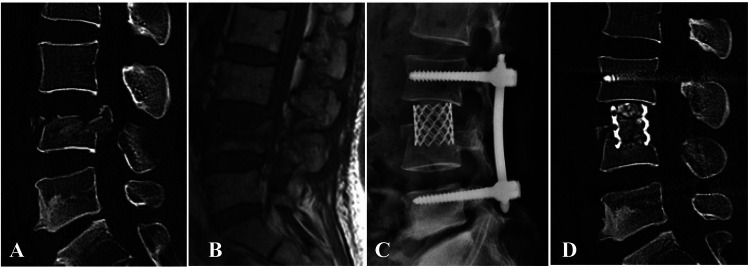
A patient with L4 burst fracture underwent posterior instrumentation combined with AIPA monosegmental reconstruction, in which the inferior endplate and disc below were preserved. (A,B) Preoperative sagittal CT scan and MRI images. (C,D) Postoperative x-ray and CT image demonstrating good correction of kyphotic deformity via posterior instrumentation combined with anterior decompression and titanium cage placement.
